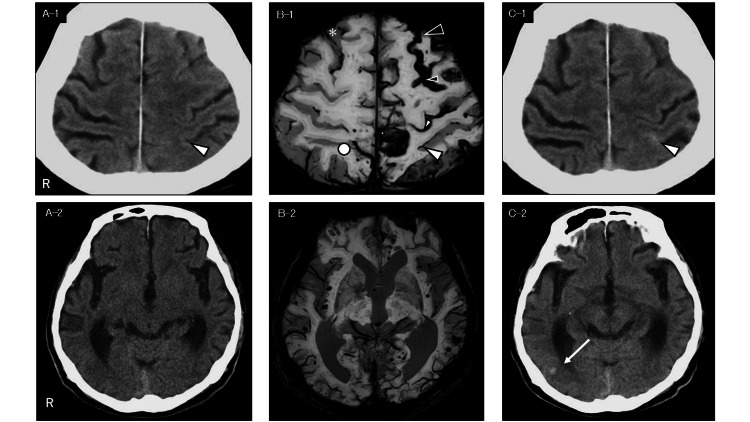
Imaging from the initial visit and the seventh month post presentation A-1, A-2: Initial CT revealed diffuse cerebral atrophy; B-1: The SWI shows acute cSAH in the left superior frontal sulcus and a thin, discontinuous hypointense line in the left postcentral sulcus. A previously unrecognized cSAH was also identified in the right parietal lobe (white circle); B-2: Bilateral CMBs were noted on SWI; C-1, C-2: The CT at stroke onset showed hyperdense lesions in the left postcentral sulcus with ill-defined margins and an ovoid hyperdensity in the right occipital lobe White arrowhead: Left postcentral sulcus; Black arrowhead: Anterior portion of the left superior frontal sulcus; Small black arrowhead: Posterior portion of the left superior frontal sulcus; Small white arrowhead: Left central sulcus; White asterisk: Anterior portion of the right superior frontal sulcus; White arrow: Hemorrhagic foci in the right occipital lobe; Symbols are consistent across A–1 to C–2. SWI: Susceptibility-weighted imaging; cSAH: Convexity subarachnoid hemorrhage; CMB: Cerebral microbleed
